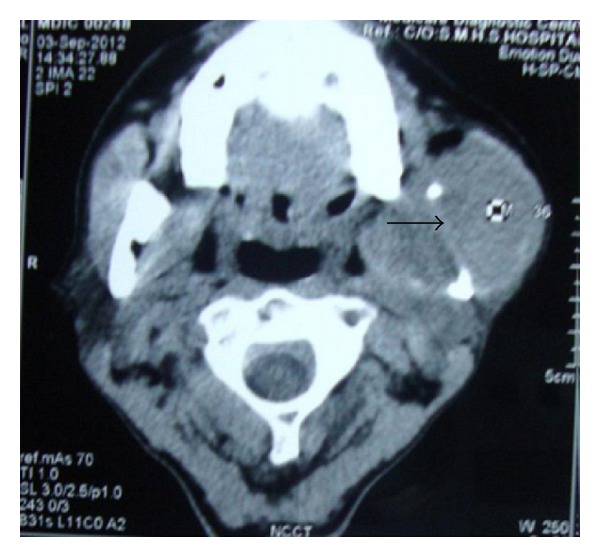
CT parotid region depicting osteolytic lesion in ramus and posterior body of mandible on left side.
